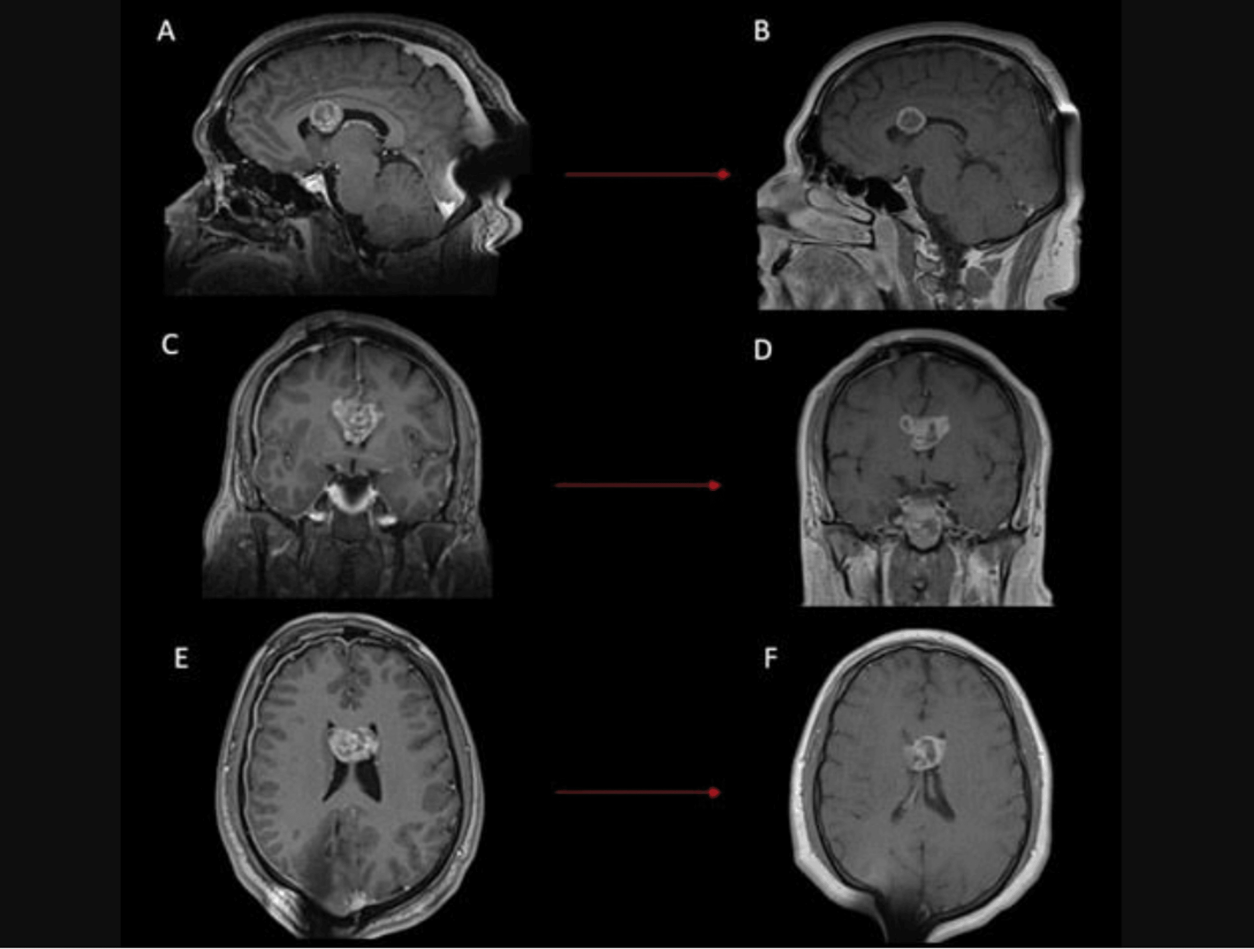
MRI Comparison Before and After Gamma Knife Treatment Showing Lesion Volume Reduction Brain MRI comparing pre-treatment imaging with the 3-month follow-up. (A) and (B) show the sagittal view, (C) and (D) the coronal view, and (E) and (F) the axial view. The images demonstrate a reduction in lesion volume along with central necrosis.
